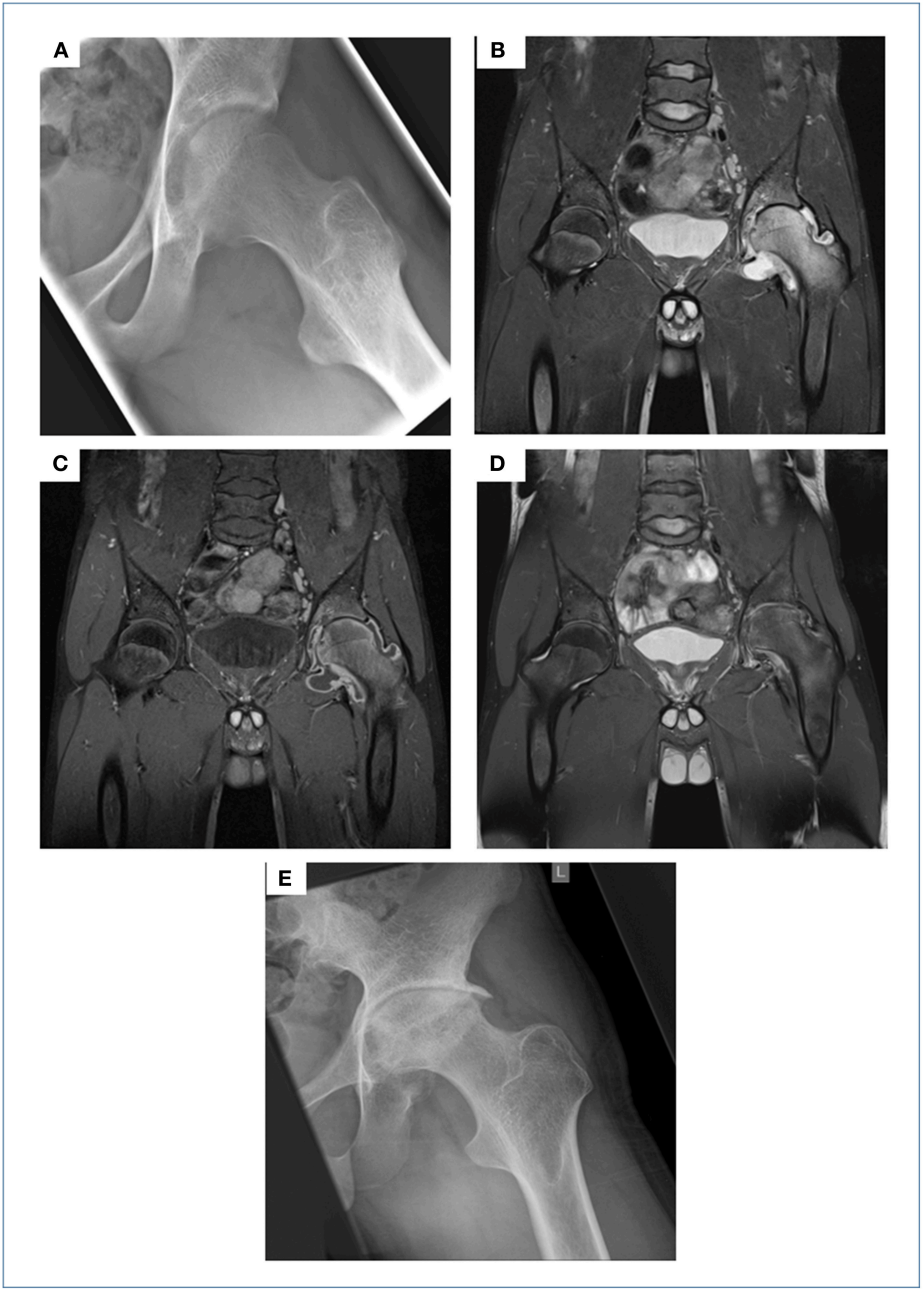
Initial X-ray of the left hip showed blurring of the cortical margins of the femoral head and acetabulum, slight demineralization, and a bulging fat pad surrounding the hip suggesting a joint effusion (A). On the initial MRI scans there was a hyper intensity of the left hip and acetabulum (=oedema of the bone marrow) as well as marked joint effusion on the T2 weighted image with fat suppression (B), synovial thickening and enhancement on the post-contrast T1 weighted image with fat suppression (C). These MRI findings were receding following 13 months of anti-TB treatment (T2 weighted image with fat suppression, D) on the one hand, but on the other hand a severe narrowing of the joint space, osteophytes and severe deformity of the femoral head became present. These findings of secondary osteoarthrosis were also demonstrated on the follow-up X-ray few months later (E).
